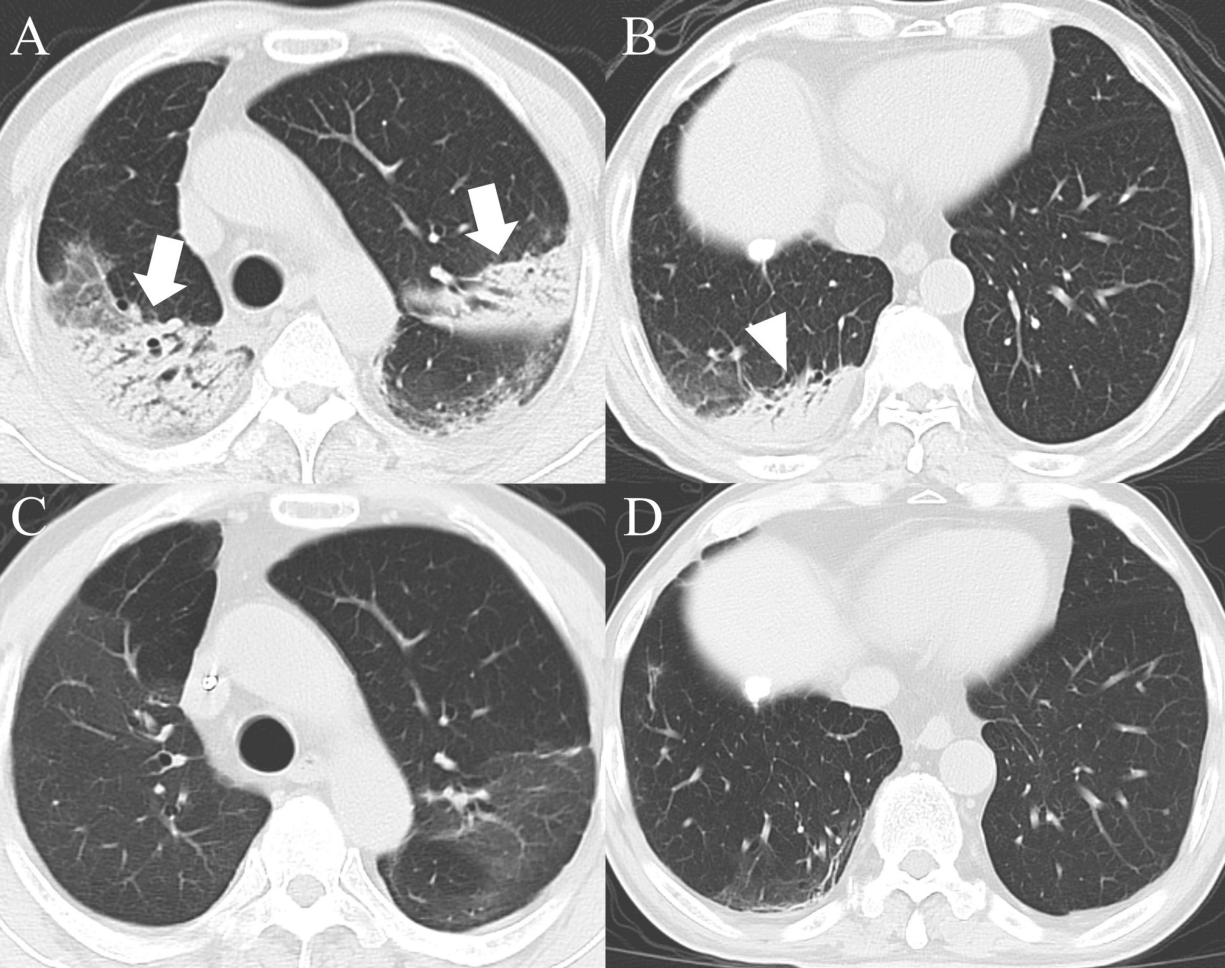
Chest computed tomography. The chest CT showed multiple bilateral consolidations during the first admission (A, arrows; B, arrowhead). No recurrence of the lung lesions was observed on the second admission (C, D).
